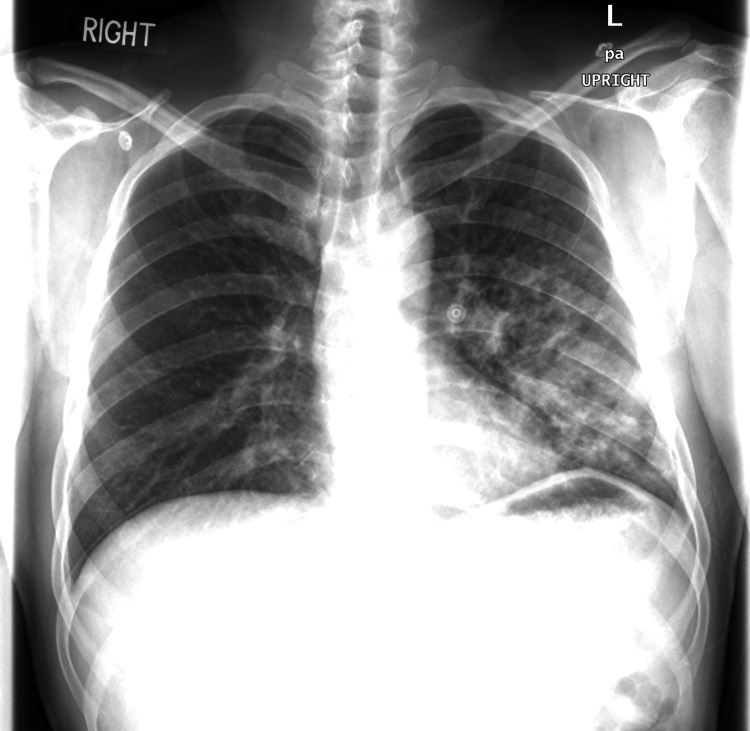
Chest X-ray showing parenchymal lung opacities in left mid and lower zones.
